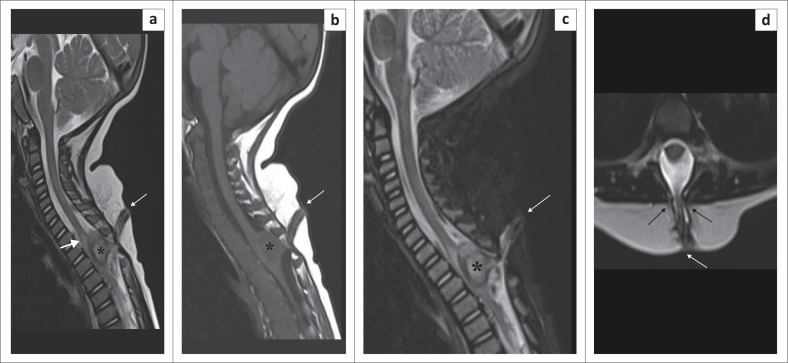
Dorsal dermal sinus. Sagittal T2-weighted image (T2WI) (a), T1WI (b), short tau inversion recovery (STIR) (c) and axial T2WI (d) show a sinus track (thin white arrow) extending from the skin surface to the thoracic spinal cord through a posterior bony defect (thin black arrows in d) at the T3 vertebral level. A heterogeneous lobulated intradural extramedullary collection (*) is causing significant spinal cord compression with associated spinal cord oedema/myelomalacia (solid white arrow).
